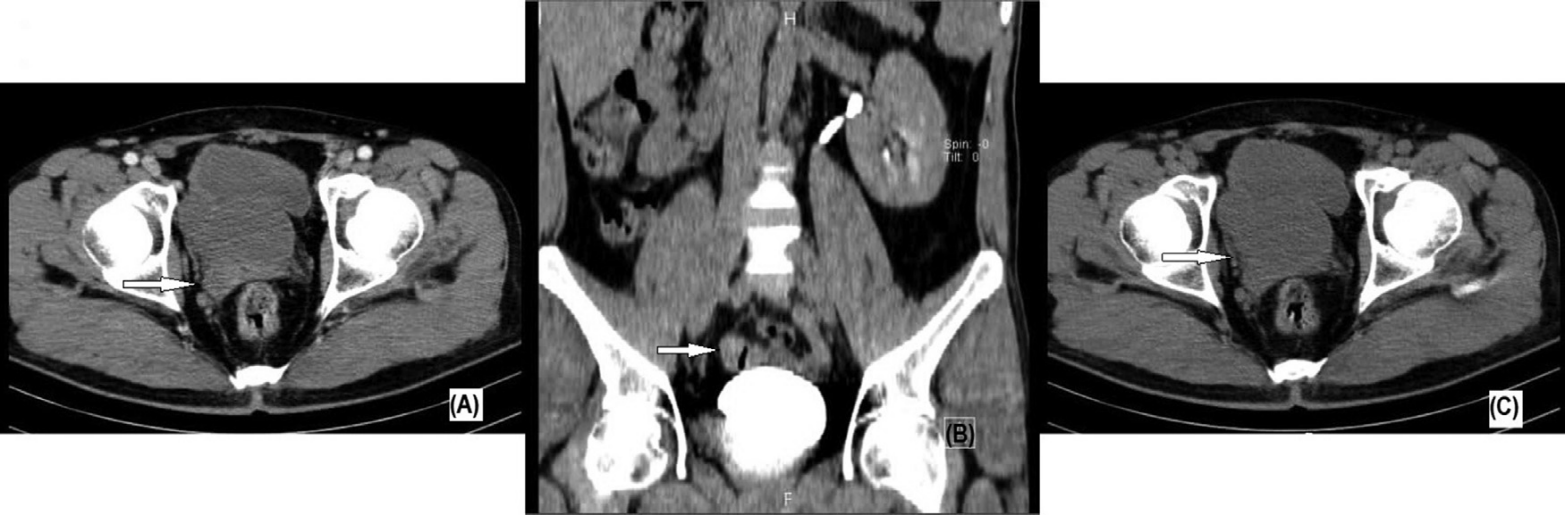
Axial (C), coronal (B), and postcontrast axial (A) CT urography demonstrating right seminal vesicle cystic changes (white arrow).
